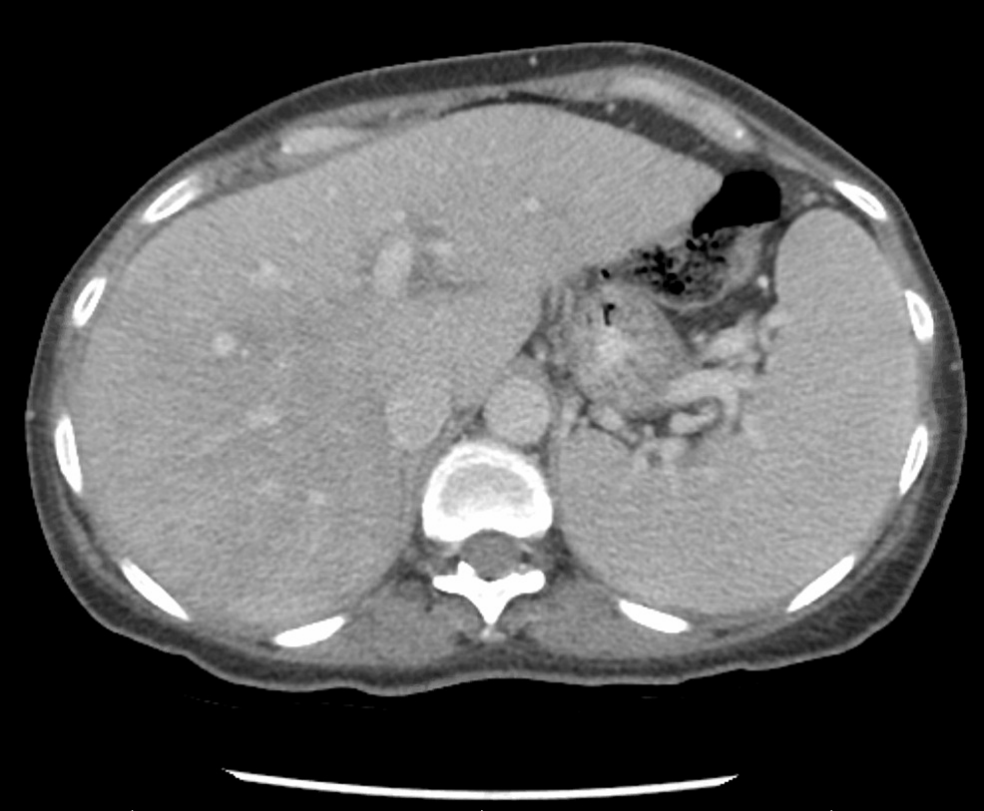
Case one: computed tomography of the abdomen and pelvis. Findings compatible with cirrhotic liver with secondary features of portal hypertension such as splenomegaly, dilated portal and splenic veins, and minimal ascites
